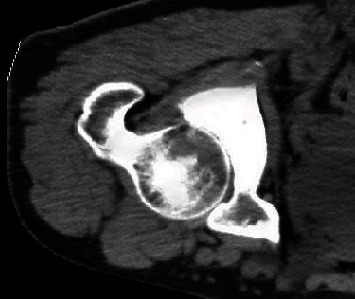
CT image of the left hip of a 60-year-old man.
